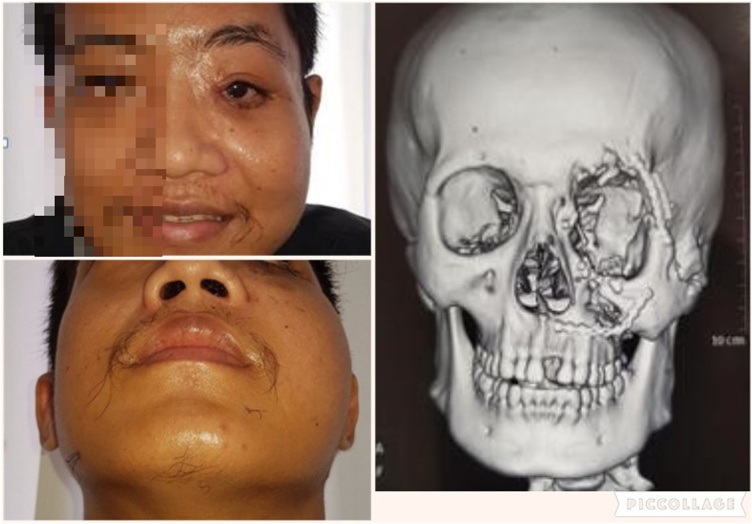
The patient’s physical appearance and three-dimensional CT-scan after reconstruction.
